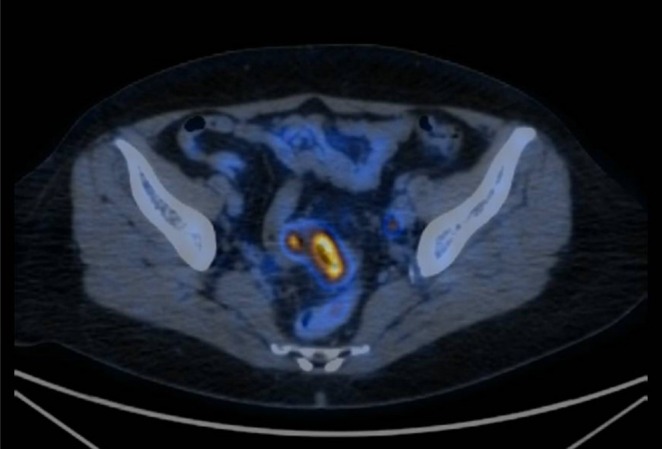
The FDG PET showing rectosigmoid junction colorectal cancer and local lymph node involvement. Clinical staging: CT3–4 N+ M1.
